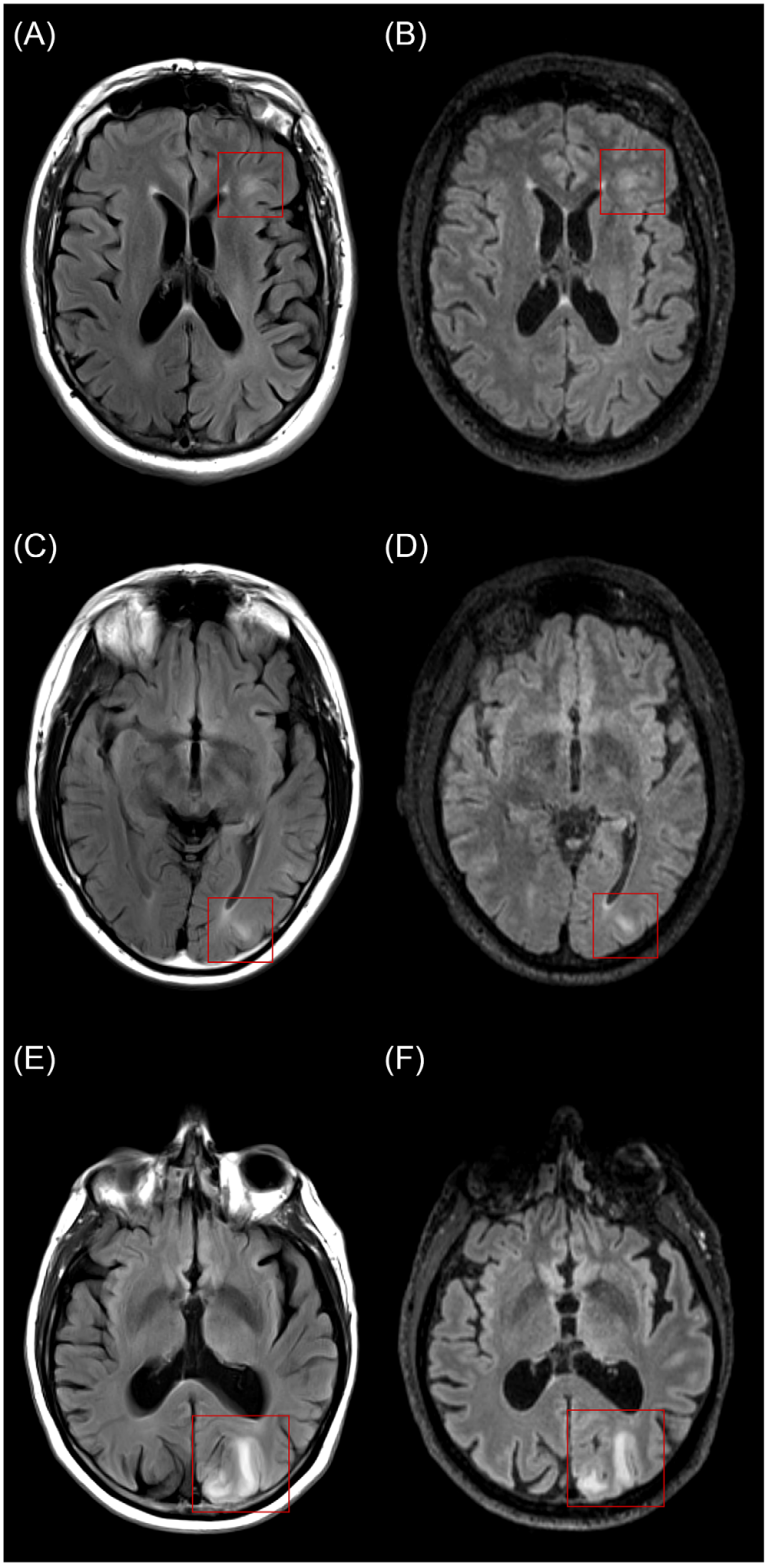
ARIA‐E. Panels (A), (C), and (E) show standard 2D FLAIR acquisitions (spatial resolution = 0.9 × 0.9 × 5mm^3^), and panels (B), (D), and (F) show fast 3D FLAIR acquisitions (spatial resolution = 1.1 × 1.1 × 1.1mm^3^). ARIA‐E regions are indicated by red squares. 2D, two‐dimensional; 3D, three‐dimensional; ARIA‐E, amyloid‐related imaging abnormalities with edema/effusion; FLAIR, fluid‐attenuated inversion recovery.
